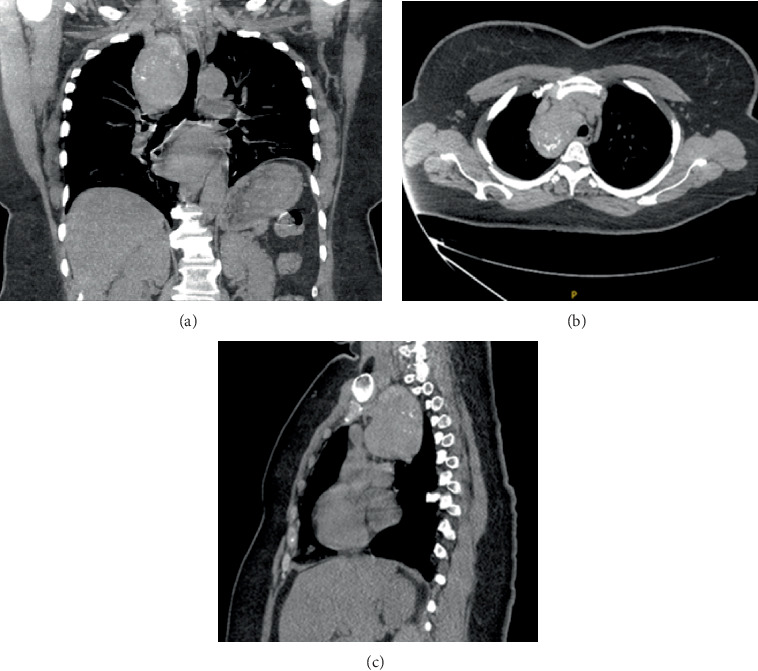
CT chest without contrast. Coronal (a), axial (b), and sagittal (c). Right paratracheal posterior mediastinum soft tissue mass 7.5 × 6.6 × 5.2 cm with few coarse calcifications and overall heterogenous density. The lesion displaces the trachea to the left.
